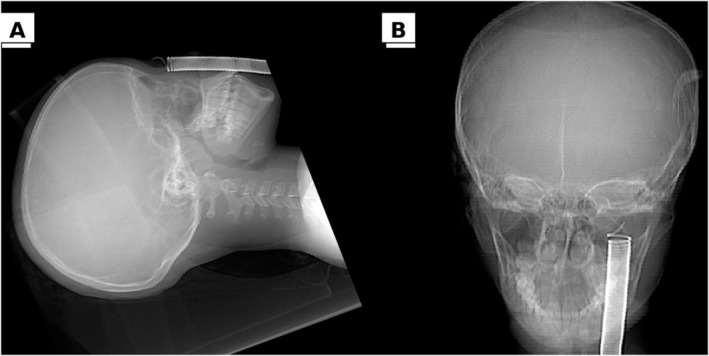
Computed tomography scout images: (A) lateral view; (B) anteroposterior view, demonstrating the coiled spring and hooked tip located anterior to the left orbit.
